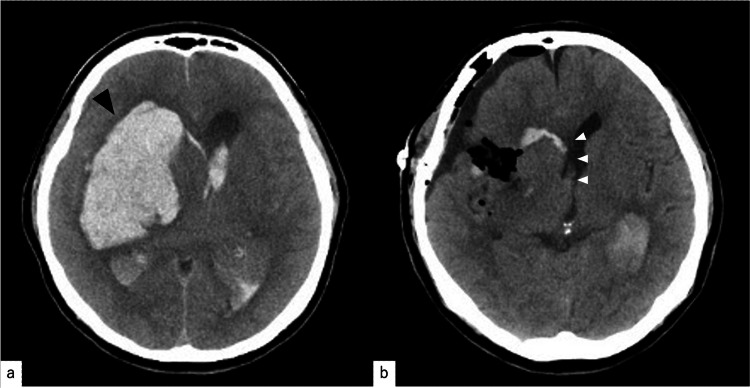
(a) Initial computed tomography (CT) in Case one: hemorrhage (measuring 8.5 × 5.3 × 4 cm in diameter with a volume of 90.1 mL) in the right basal ganglia region (black arrow). (b) Postoperative CT: almost complete removal of the lesion with a significant reduction in the midline shift (white arrow).
